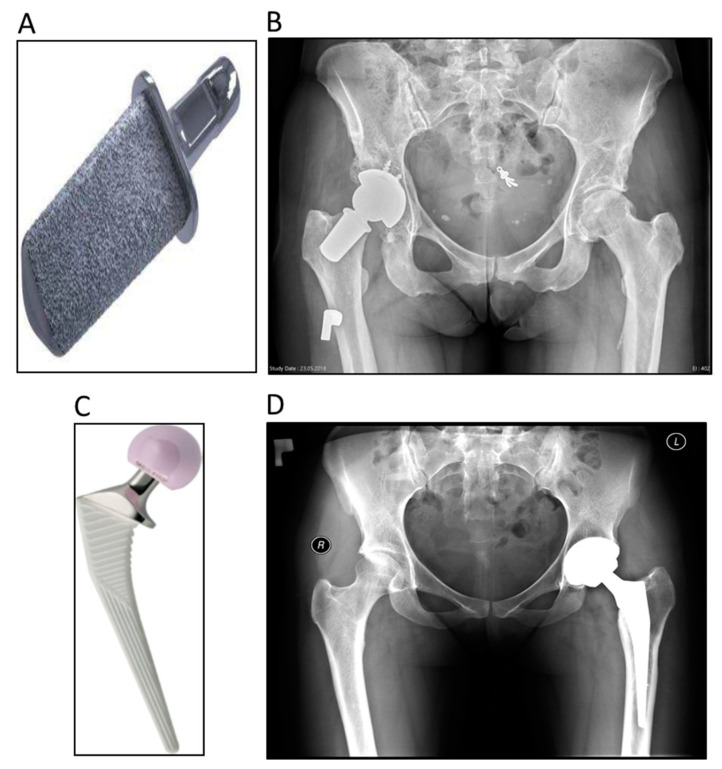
The femoral neck prosthesis (Biomet information materials) (A) and postoperative radiograph (B). The conventional hip stem system (DePuy information materials) (C) and postoperative radiograph (D).
